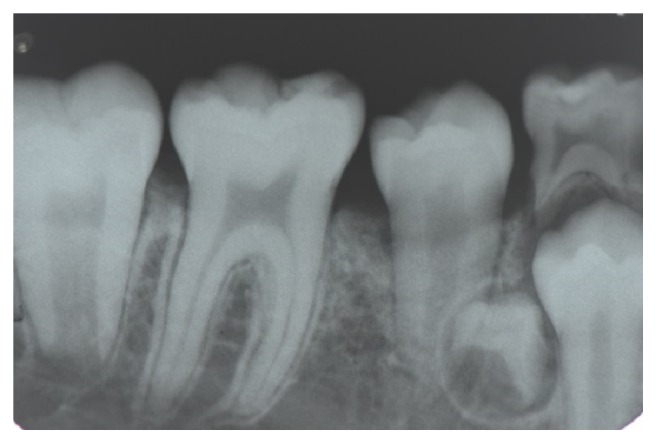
Periapical radiograph of the patient aged 9 years and 10 months showing early formation of a supernumerary tooth in the right mandibular premolar region.
